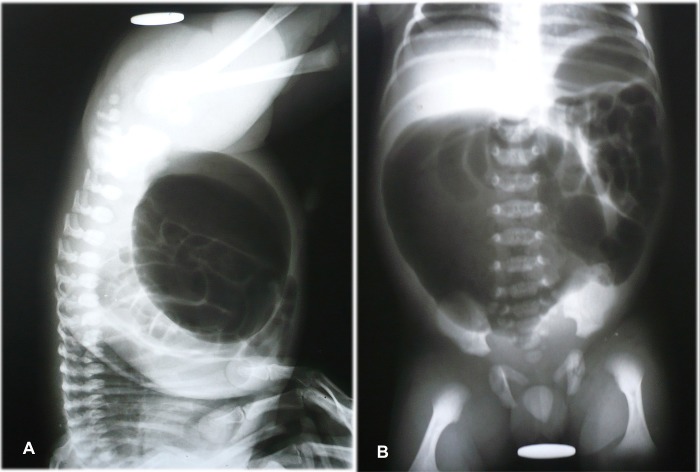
Figure 1: (A) Abdominal radiograph (invertogram) showing a large loop of bowel filled with meconium and air, occupying more than half of the abdominal cavity. (B) Erect radiograph of the same patient.
